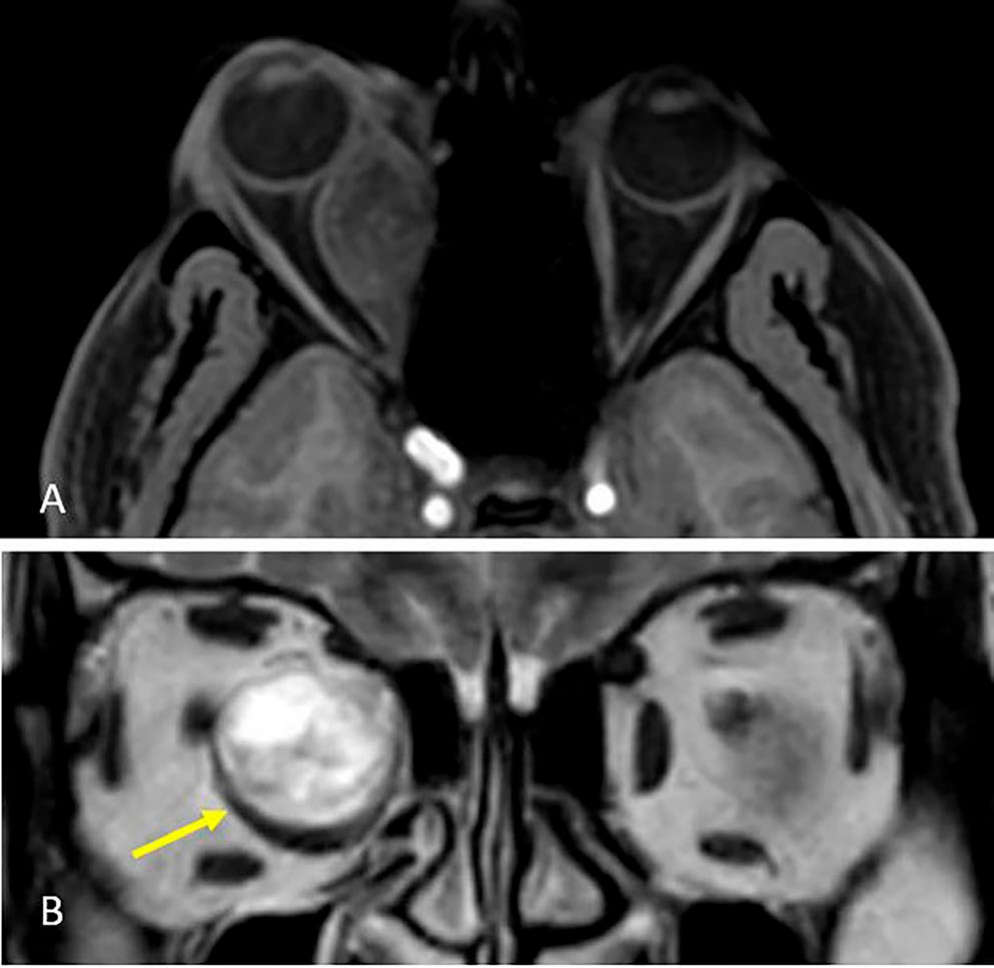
MRI. (A) T1‐weighted with contrast showed a large orbital mass heterogeneously enhancement and cystic space. (B) T2WI showed large hyperintense mass displace medial rectus (yellow arrow).
